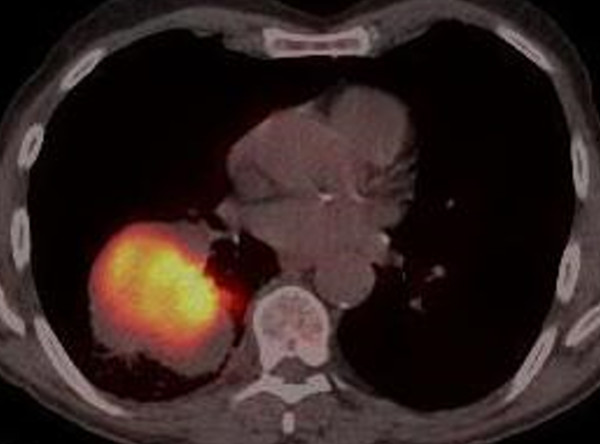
A positron emission tomography-computed tomography (PET-CT) demonstrated increased fludeoxyglucose uptake within the mass, standardized uptake value (SUV max22.1) and no evidence of nodal involvement or distant metastases.
